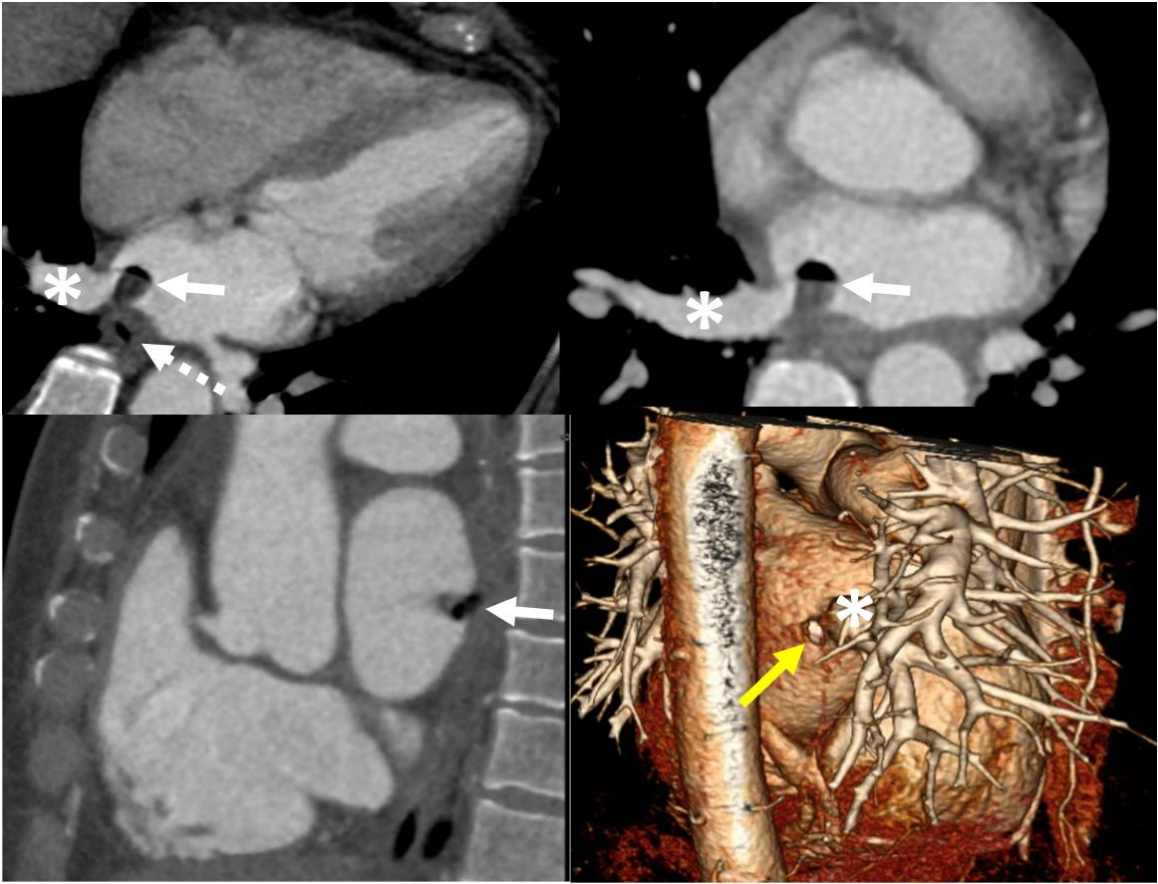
(Above) Contrast-enhanced axial chest computed tomography showing a filling defect in the left atrium with a small air bubble (white arrow) located at the base of the right inferior pulmonary vein (asterisk) with a fistulous tract connecting this filling defect to the oesophagus (dashed arrow). (Below left) A gated sagittal heart shows the same findings. (Below right) A volume-rendered three-dimensional image of the heart depicts the fistula in the posterior left atrium wall (yellow arrow), adjacent to the right inferior pulmonary vein (asterisk).
